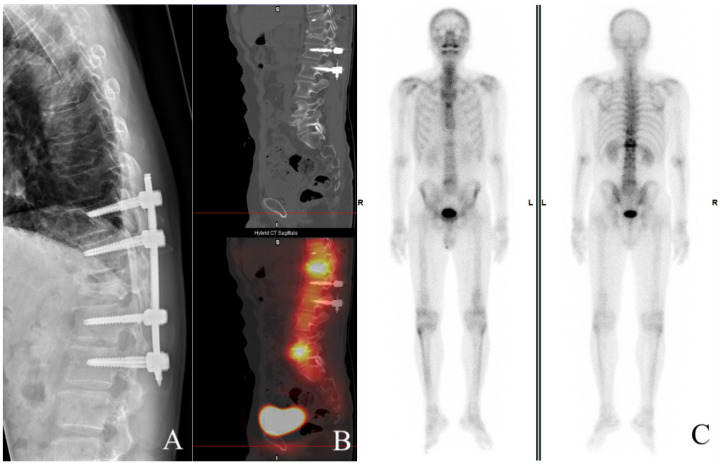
Postoperative follow-up at 4 months (A–C). X-ray documents transpedicular screw fixation of T10,11-L1,2 with posterior-lateral fusion. Obvious compression of T12 appears (A) and increased uptake, is shown on both SPECT (B) and whole body bone scan (C), respectively.
